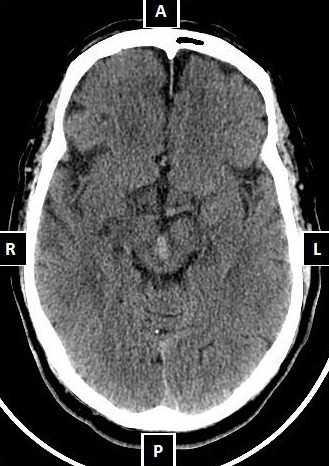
Cerebral computed tomography (CT) scan showing a spontaneous hyperdensity lesion of the midbrain consistent with a midbrain hematoma.
